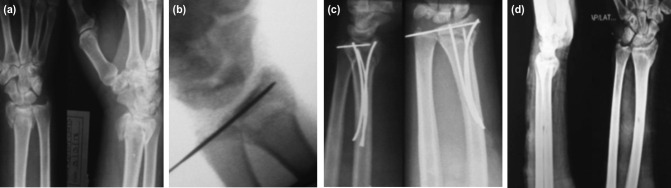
Radiographic images showing series of pre-operative to post-operative progression for one patient without the use of external fixation; (a) images showing comminuted distal radial fracture with instability of the DRUJ, (b) K-wire pinning across the DRUJ, (c) post-operative images following fixation, (d) and AP and lateral images at final follow-up showing radiographic union.
